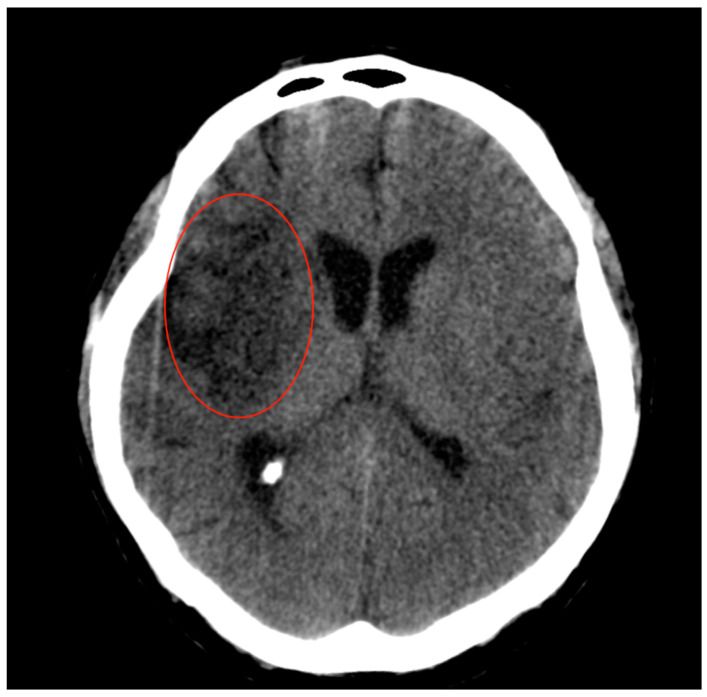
Head CT scan perform at 7 days after MT showed ischemia in the vascularized territory (lentiform nucleus and in the fronto-temporal-insular right lobe) (red circle).
